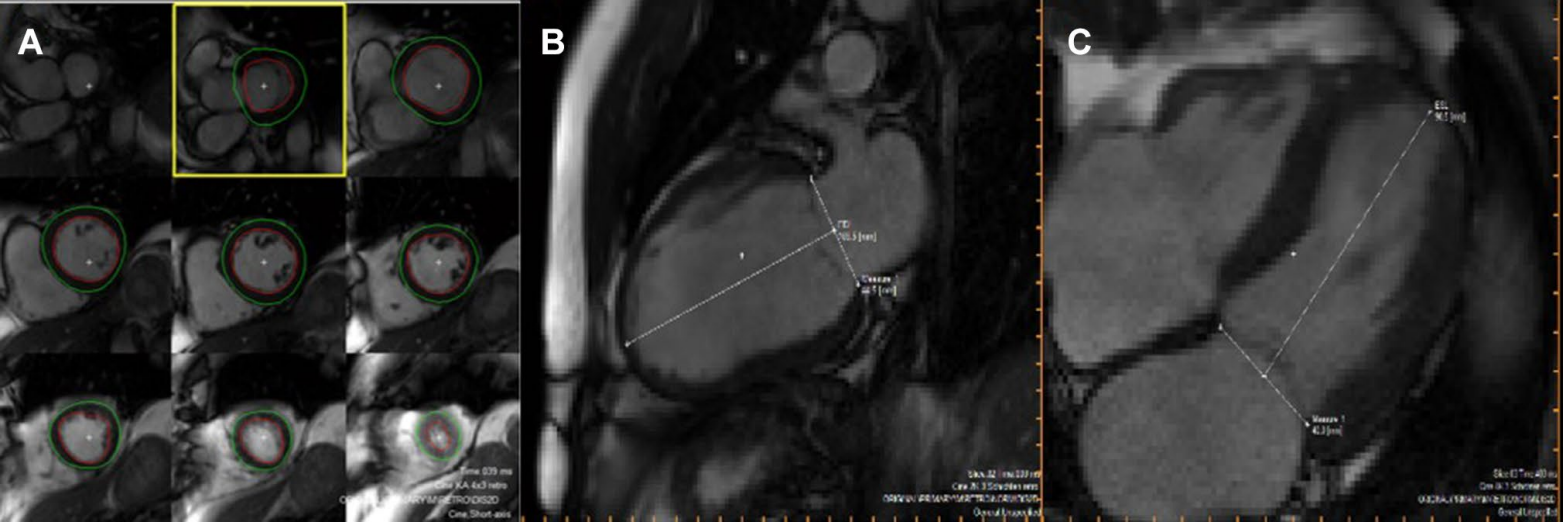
Example of magnetic resonance imaging of the left ventricle: a Planimetry, b, c size of left ventricle
